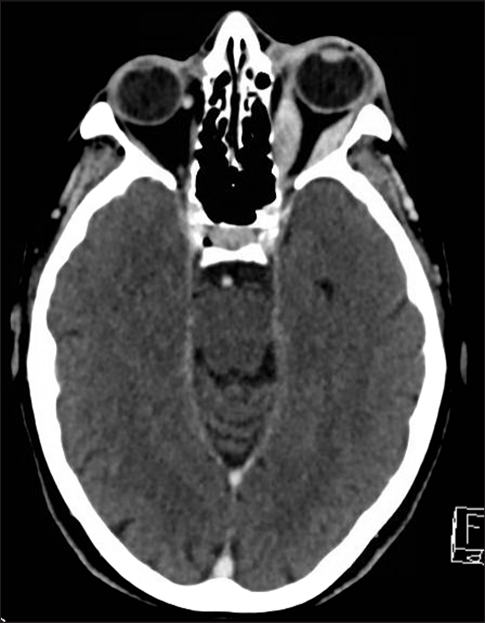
Contrast-enhanced axial CT of the brain shows fusiform and contrast-enhancing thickening of the left lateral and medial rectus muscles.
